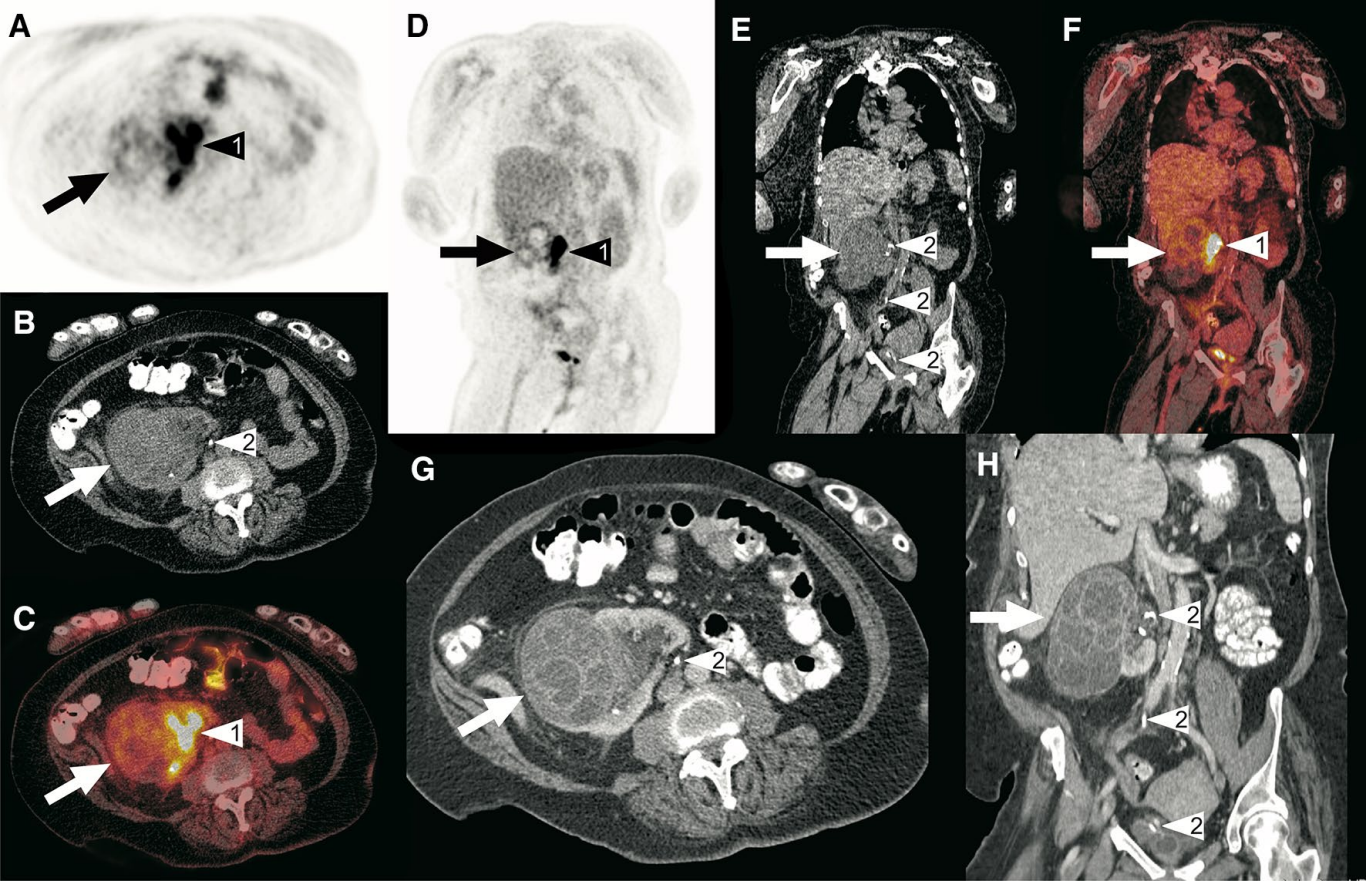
A 62-year-old woman was admitted to the department of internal medicine because of abdominal pain and fever. A couple of weeks before, she was diagnosed with a large obstructing right ureteral stone, for which a double-J catheter was placed. Blood and urine samples were taken, which both tested positive for *Proteus mirabilis*. The CRP level was 65 mg/L and the white blood cell count was 12.4 × 10^9^/L. The working diagnosis was urosepsis and the patient was started on ciprofloxacin and amoxicillin/clavulanic acid, which was later switched to meropenem. Despite antibiotic treatment, high infectious parameters (CRP level of 101 mg/L and white blood cell count of 13.8 × 10^9^/L) and fever remained. To assess for any underlying cause of infection, an FDG-PET/CT scan was ordered. Axial and coronal FDG-PET (a, d), low-dose CT (b, e) and fused FDG-PET/CT (c, f) show an enlarged contour of the right kidney that appeared cystic, but with only slightly increased FDG uptake (arrows). Because of the slightly increased FDG uptake, infection was deemed unlikely. No pathologic FDG-avid foci were detected elsewhere. Axial (g) and h portal-venous phase full-dose CT scans showed a multi-cystic lesion in the cortex of the right kidney (arrows), with a differential diagnosis that included cyst infection and cystic neoplasm. Also note normal pyelocaliceal FDG accumulation in the right kidney (arrowheads no. 1 in a, c, d, and f) and the double-J catheter (arrowheads no. 2 in b, e, g, and h). Because the patient had already been treated with high-dose intravenous antibiotics for a long time without clinical improvement, a nephrectomy was performed, which demonstrated a necrotizing and suppurative infection of multiple cysts, without any signs of malignancy. The patient was discharged from the hospital in good clinical condition shortly afterwards
